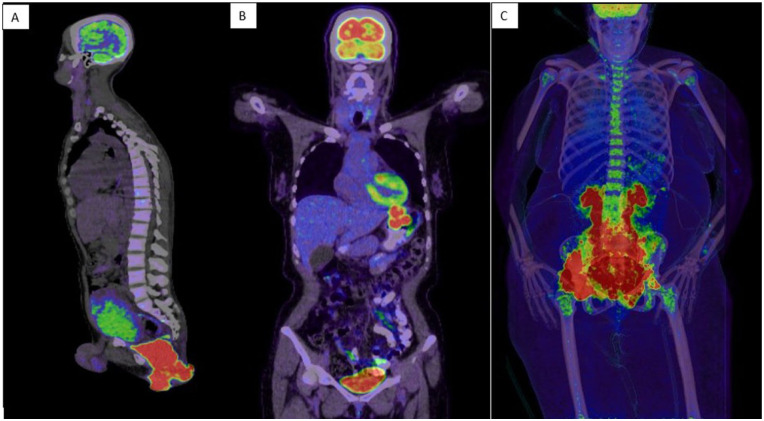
(a) FDG PET/CT imaging revealed an intensely hypermetabolic anorectal mass corresponding to the biopsy-proven lymphoma, accompanied by an intensely hypermetabolic perirectal lymph node. The scan also demonstrated innumerable osseous foci suspicious for lymphomatous infiltration, resulting in Stage IV disease classification. (b) FDG PET/CT staging demonstrated Stages II–E disease with isolated gastric involvement. (c) FDG PET/CT showed a large retroperitoneal enhancing mass extending throughout the pelvic cavity and lower abdomen, with extension from the ischiorectal fossa to the right gluteus muscles. This mass caused significant compression of surrounding structures, notably including bilateral ureteral encasement with resultant hydroureteronephrosis. CT: computerized tomography.
